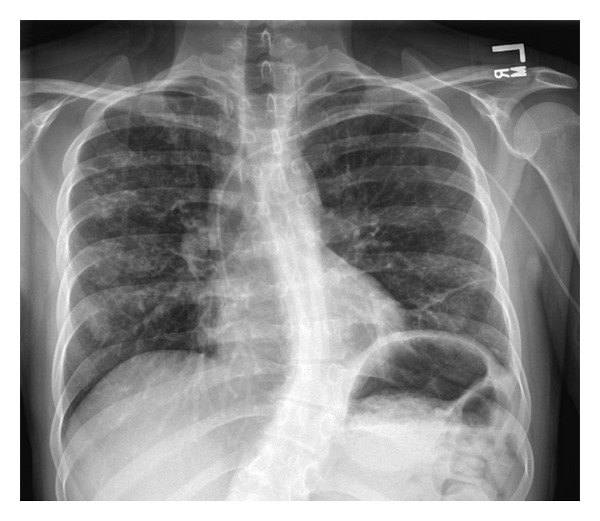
Chest radiograph taken two weeks after discharge from the hospital shows marked improvement of pleural and parenchymal disease. Central line for infusion of antibiotics is visible.
